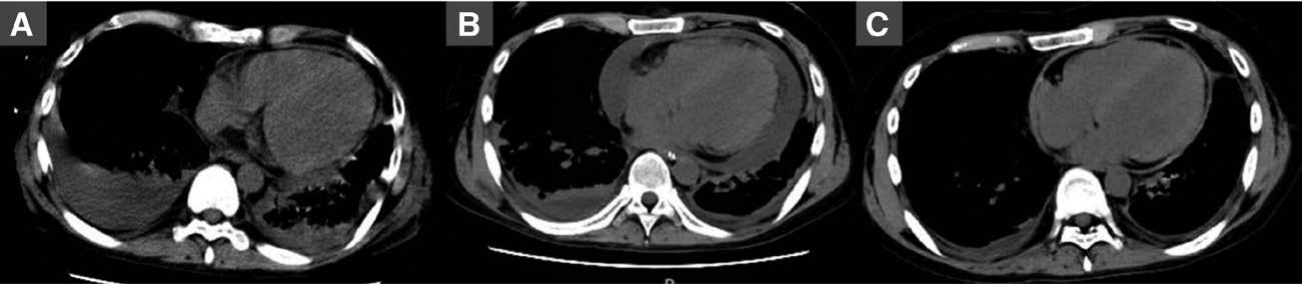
A chest computed tomography (CT) scan performed after admission to the intensive care unit (A), on the 39th d (B), and the 57th d (C). (A) CT showed pericardial effusion and pleural effusion. (B) Pericardial fluid increased. (C) Pericardial effusion improved.
